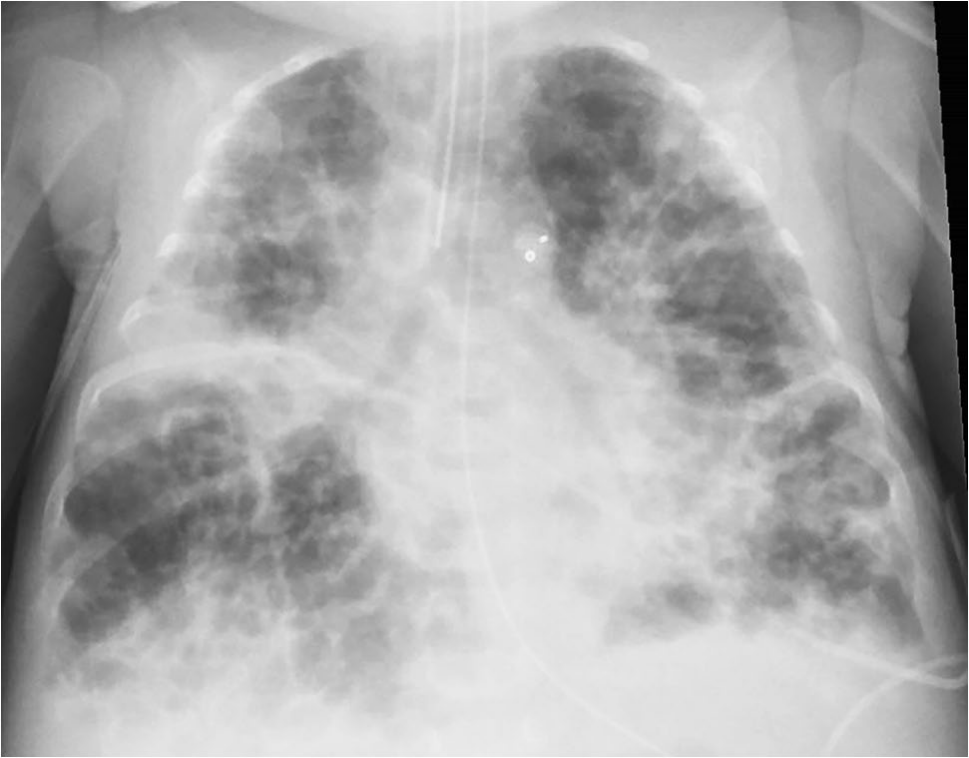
Typical appearance of bronchopulmonary dysplasia (BPD). Anteroposterior (AP) chest radiograph of a girl born at 25 weeks of gestation who is now post-menstrual age 36 weeks (11 weeks old) with severe BPD. The girl is still intubated and has a ductus arteriosus closure device. The lungs are characterized by overall hyperinflation, with mixed areas of density and hyperlucency characteristic of the AP chest radiograph appearance of severe BPD
